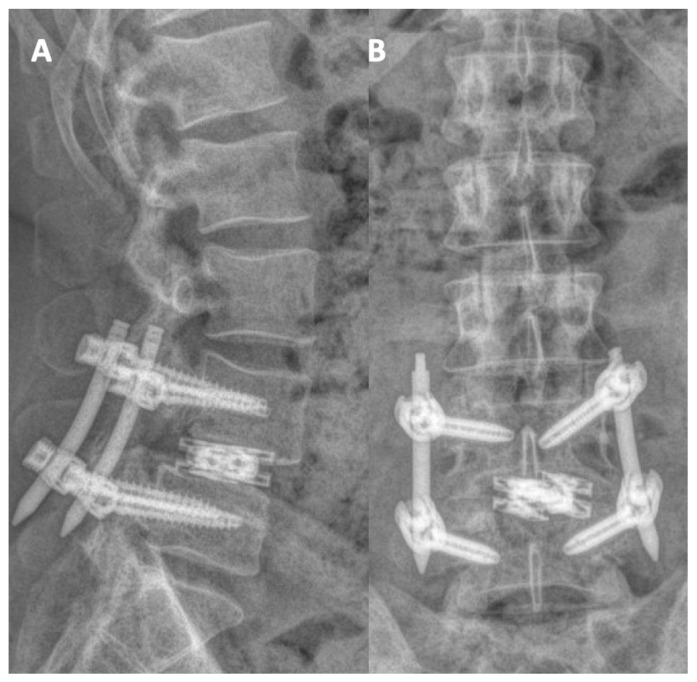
(A) AP and (B) lateral views of the postoperative standing X-rays demonstrating satisfactory reduction of the slip and reassuring hardware integrity.
